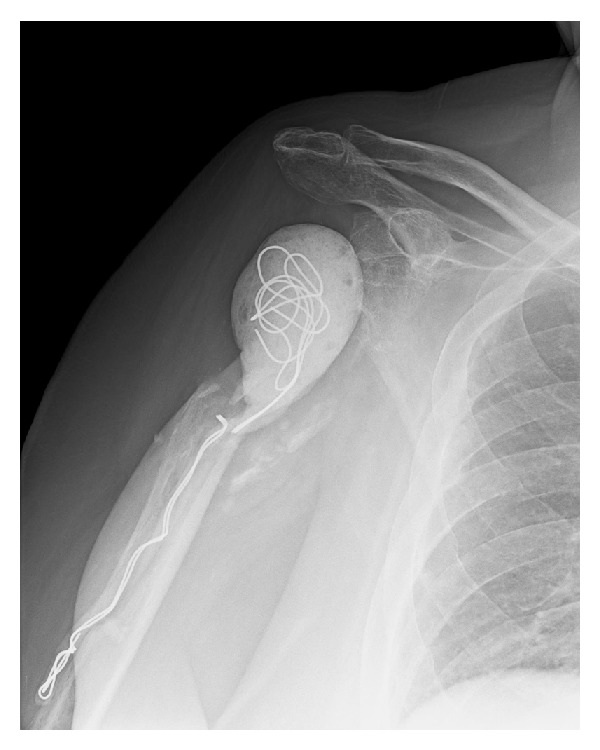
Radiograph at one year after the final I and D showing the cement spacer that the patient was asked to “live with.”
